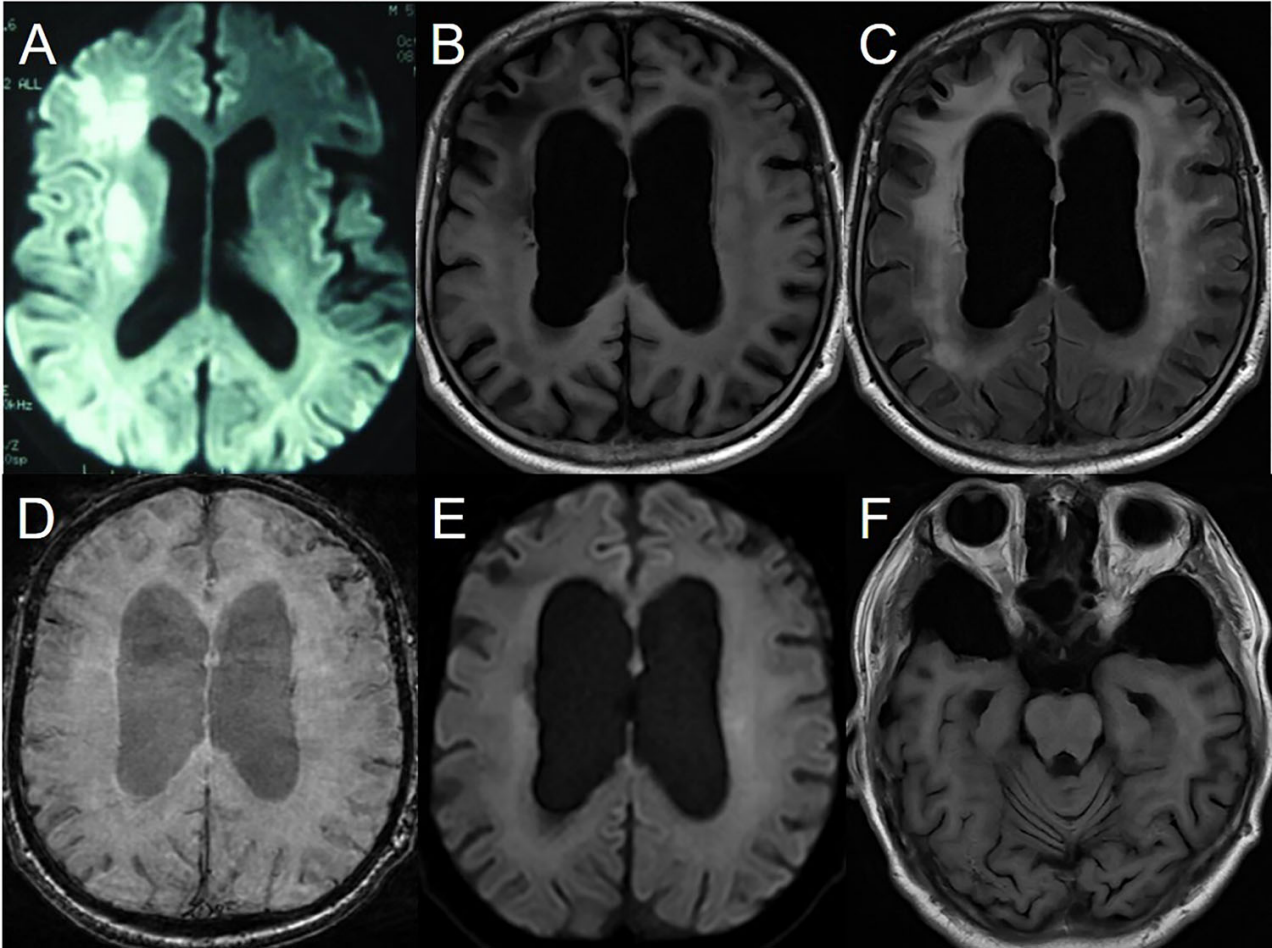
Brain imaging of Patient 2. (A) Restricted diffusion in the right frontal lobe, occipital lobe, and basal ganglion at the age of 51. (B–F) Brain magnetic resonance imaging at age 64 showed multiple subependymal nodules in lateral ventricles and septum pellucidum, predominantly at the roof level. The nodules showed isointensity on susceptibility‐weighted imaging (D) and diffusion‐weighted imaging (E). Periventricular, subcortical, and deep white matter changes were remarkable (C). U‐fibers were involved, but this could be confounded by ischemic lesions. There were multiple infarctions in the right frontal lobe, occipital lobe, and putamen (not shown). Moderate frontotemporal hypoplasia (F), brain atrophy, and ventricular enlargement were noticed.
